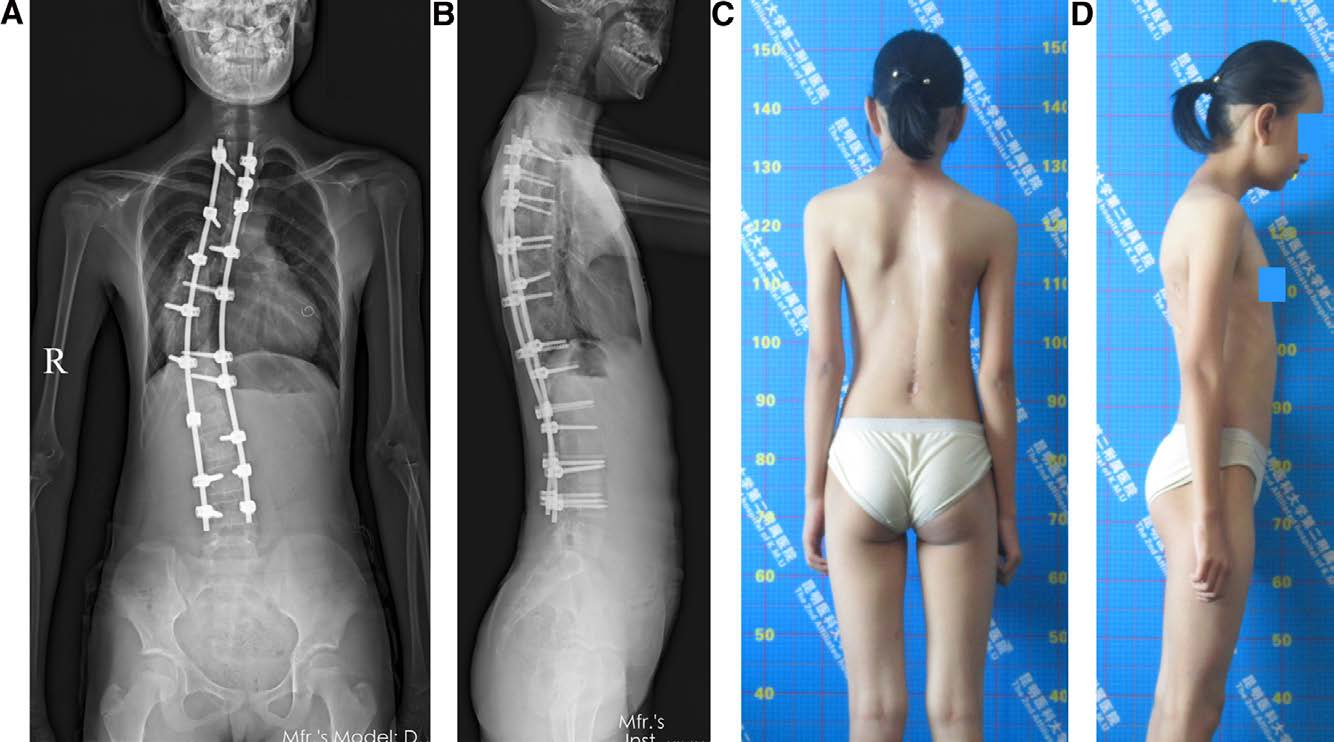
Results 26 months after surgery. (A) An AP X-ray shows a Cobb angle of the coronal main curve of 51°, RSH of 1.5 cm, and C7-CSVL of 1.17 cm. (B) On a lateral X-ray, the Cobb angle is 31°, and the SVA is 1.4 cm. (C) Posterior view. (D) Lateral view. AP = anteroposterior, C7-CSVL = C7-central sacral vertical line, RSH = radiographic shoulder height.
